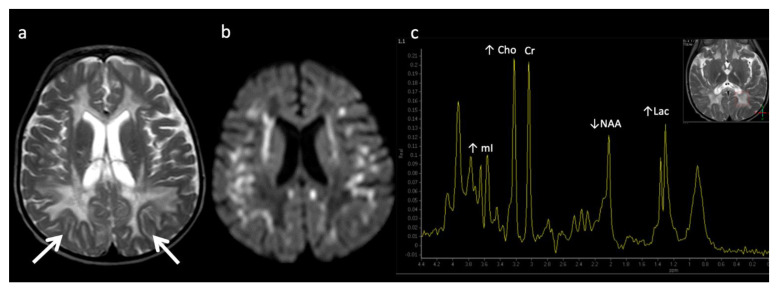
Metachromatic leukodystrophy in a 16-month-old female who presented with loss of developmental milestones. (a) Axial T2WI and (b) axial DWI demonstrate cerebral volume loss with ex vacuo ventriculomegaly, a widespread leukodystrophy with increased white matter signal sparing the U-fibers (arrows) and involving corpus callosum, and reduced diffusion at leading-edges of active demyelination. (c) SV-MRS over the left periatrial white matter shows elevated Lac, severely depleted NAA, and slightly elevated Cho and mI. Findings are consistent with considerable axonal damage and loss of the white matter.
